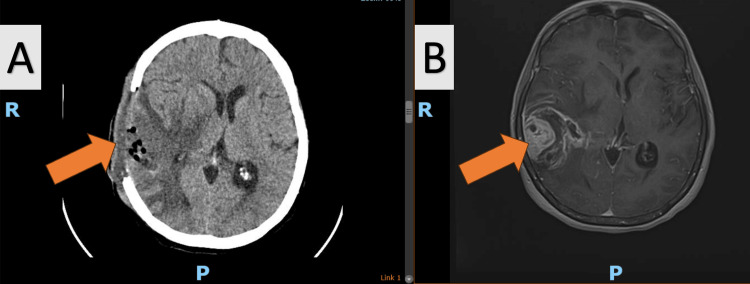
(A) Post-operative CT scan that shows tumoral resection and no active hemorrhage, (B) preoperative MRI scan that outlines the tumoral lesion
